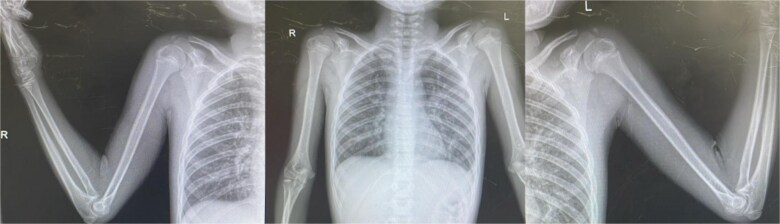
Humerus (X-ray). Radiographic (X-ray) evidence of the humerus reveals epiphyseal plates that are more developed than expected for the patient’s chronological age. This advanced bone maturation supports the diagnosis of precocious puberty and further correlates with the patient’s early physical development.
